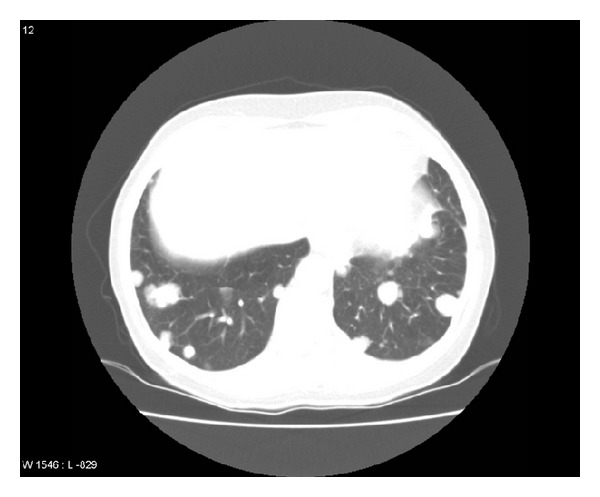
Computed tomography scan of the chest showing multiple bilateral lung metastases.
